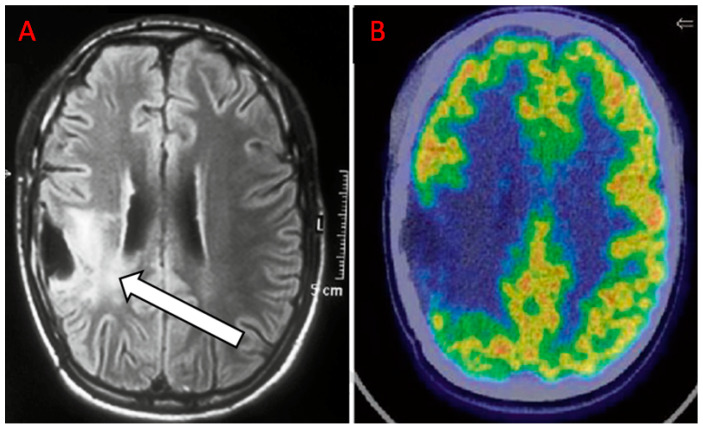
Anaplastic oligoastrocytoma treated with surgery, radiotherapy (RT), and concurrent temozolomide eight months before. (A) magnetic resonance imaging (MRI) shows suspicious findings for recurrence (white arrow). (B) the ^18^F-FDG PET/CT scan document no uptake, which is compatible with radionecrosis (RN) (Courtesy of Dr. Cistaro).
